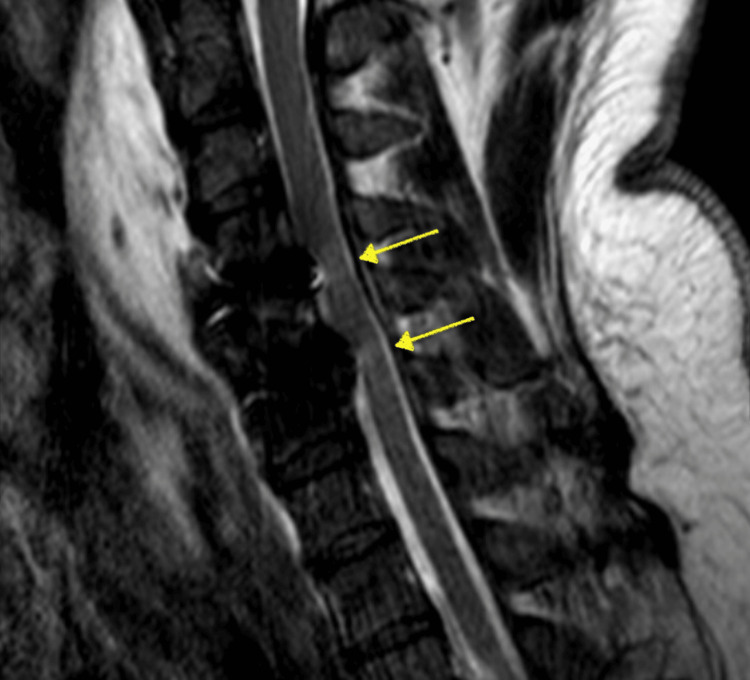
Sagittal view of a T2-weighted MRI of the cervical spine showing a hyperintense signal at the C4-C6 levels (yellow arrows).
